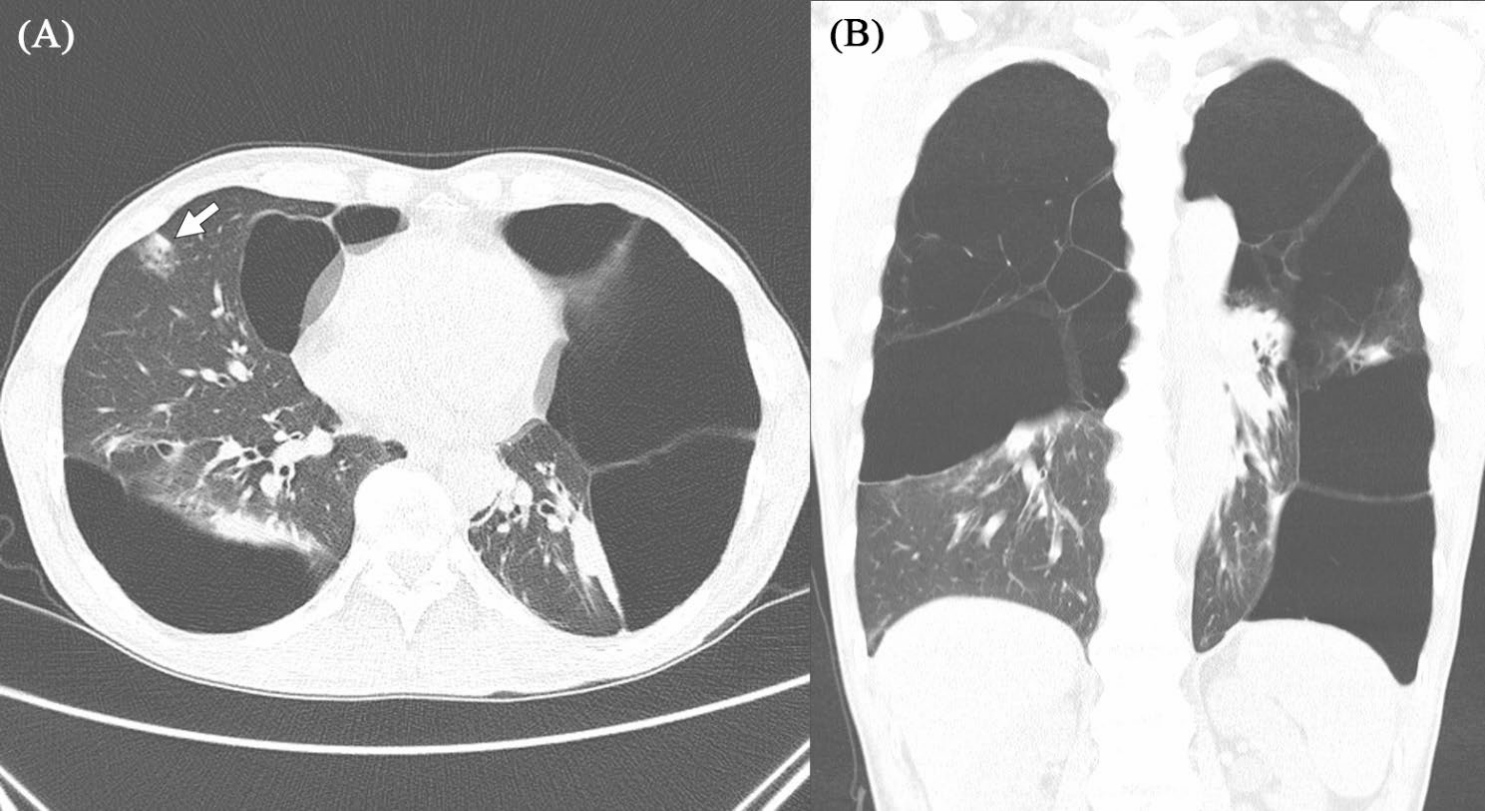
Preoperative contrast-enhanced chest CT revealed the following: (A) Giant bullae in both the hemithoraces and lung cancer in the right middle lobe (white arrow) in the axial view. (B) Giant bullous emphysema in both the hemithoraces in the coronal view. CT, computed tomography
